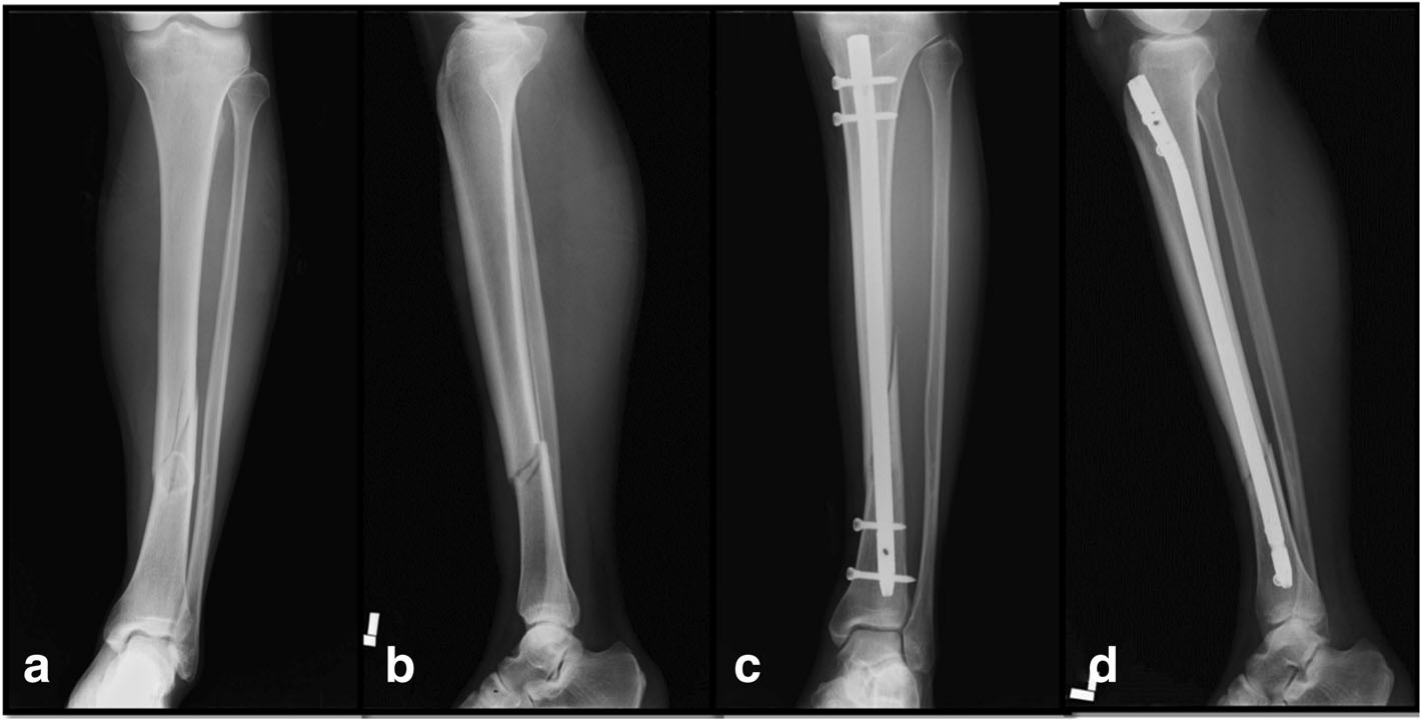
a, b Anteroposterior and lateral radiographs of a tibia fracture in a 43-year-old man. Plain films showing a fracture at the junction of the proximal and one third of the tibia. c, d Bone union following intramedullary nailing shown in anteroposterior and lateral views
